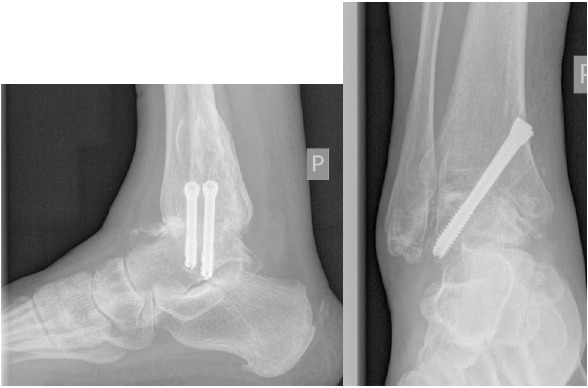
Weight bearing AP and lateral radiographs six weeks after AAA with two parallel HCS compression. Note radiographic bone union at the arthrodesis site.
